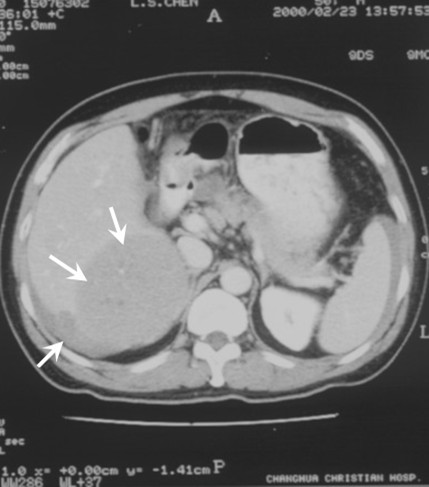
Abdominal computed tomography on admission showing a mass in Couinaud segment 7 and segment 8 of the liver and intraperitoneal fluid.
